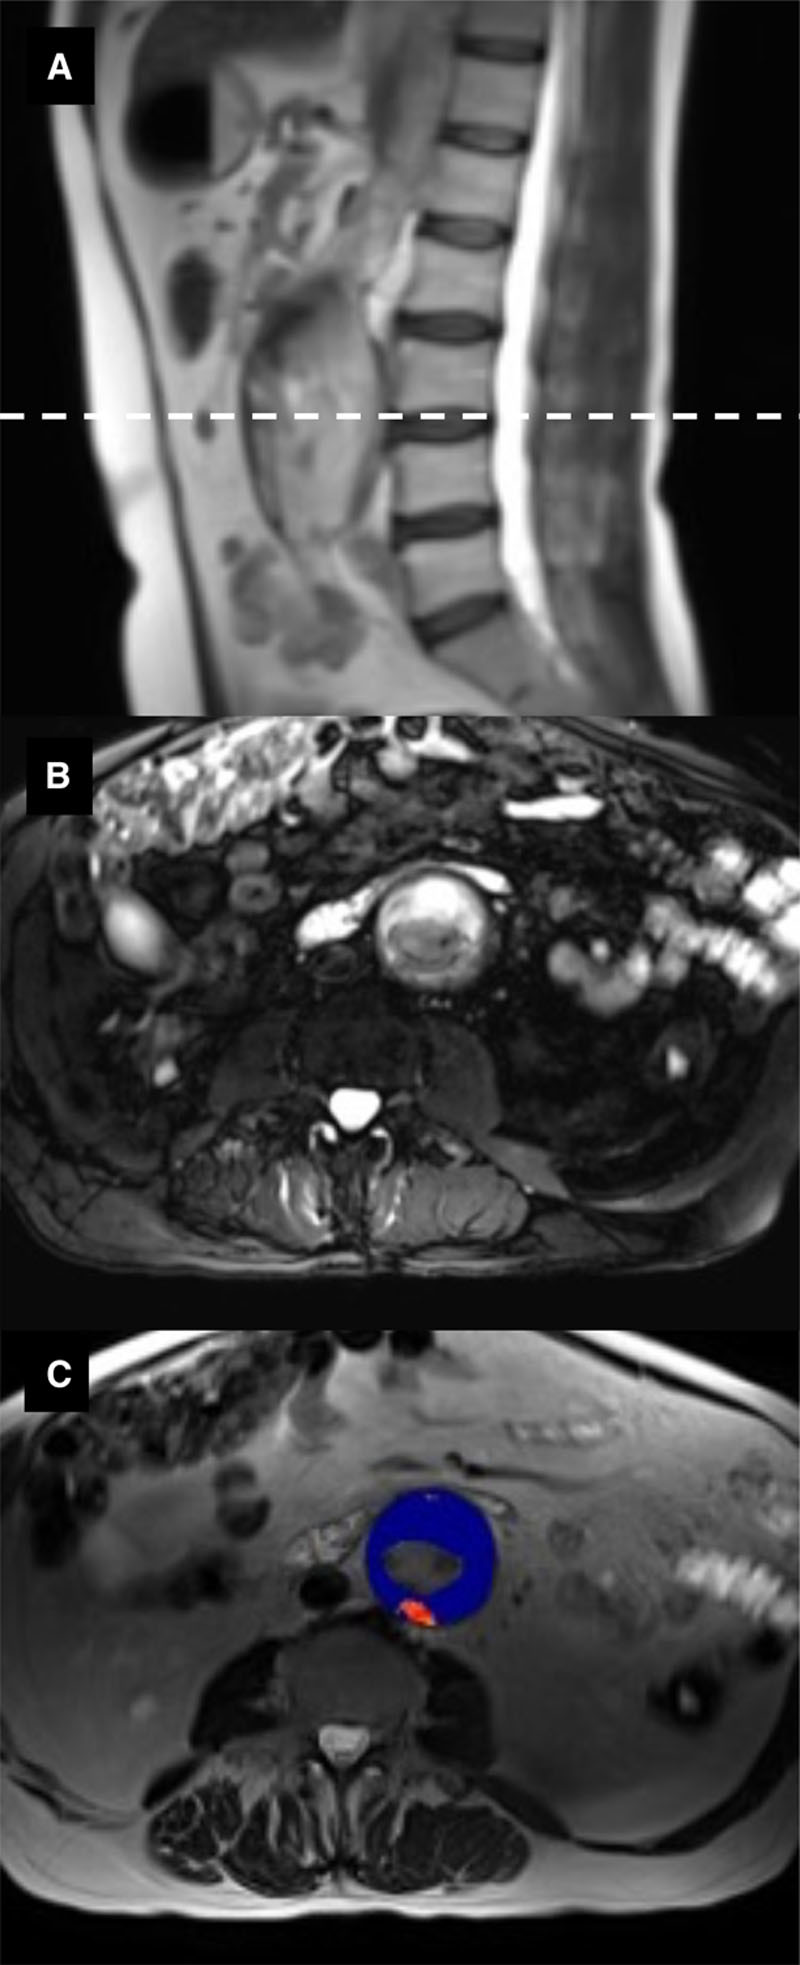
MRI of abdominal aortic aneurysm.
